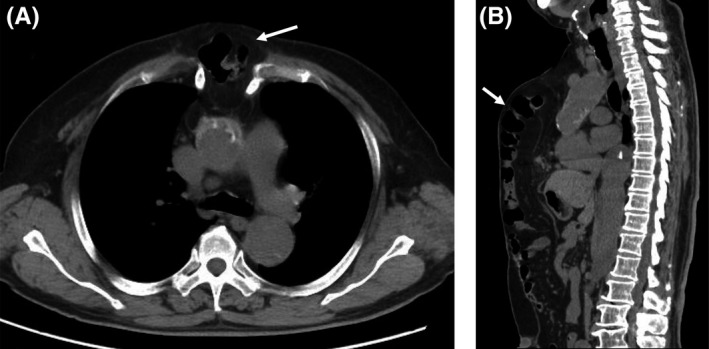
CT scan (A, axial and B, sagittal) showing herniation of the transverse colon into the mediastinum (arrow)
